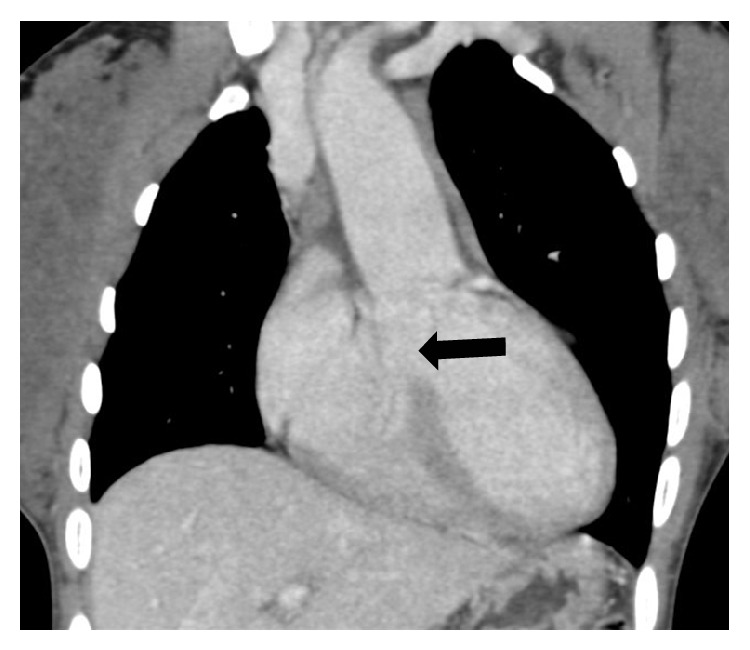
Coronal CT pulmonary angiography reveals a large subaortic VSD with overriding of the aorta (thick arrow).
